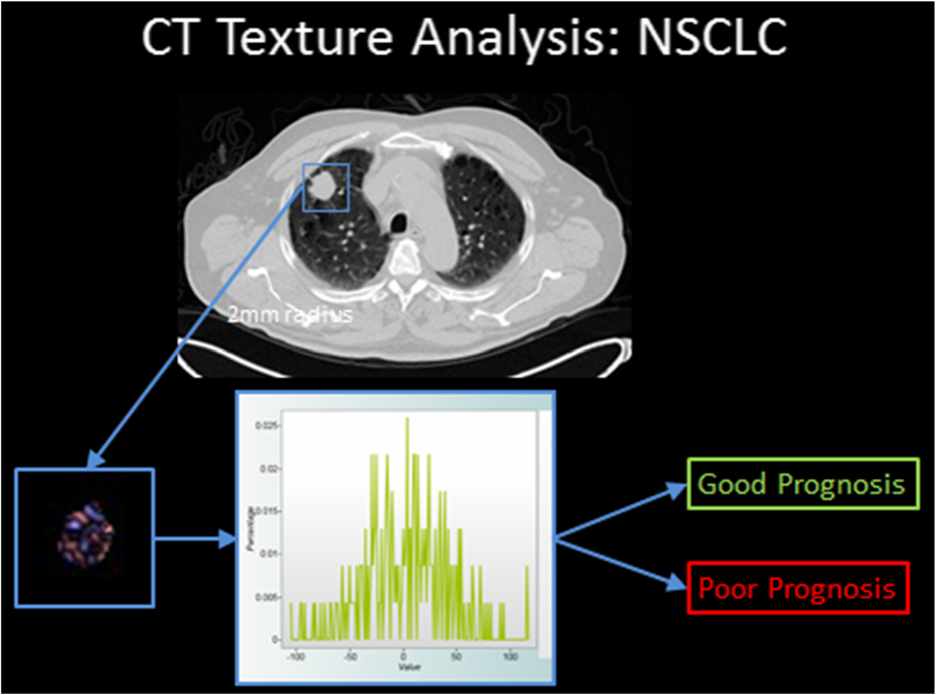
Summary of the filtration-histogram method for CTTA. The conventional CT image (top) is filtered to highlight objects of a pre-selected size. The distribution of tumour features within the filtered image as assessed using standard statistical parameters derived from the corresponding histogram, provides an indication of prognosis
